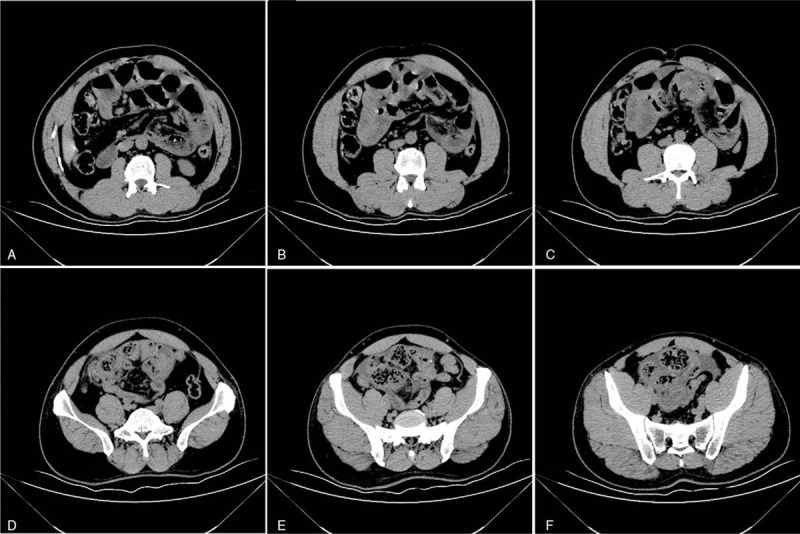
Longitudinal computed tomography images showed typical abdominal cocoon entrapment in the small intestine.
